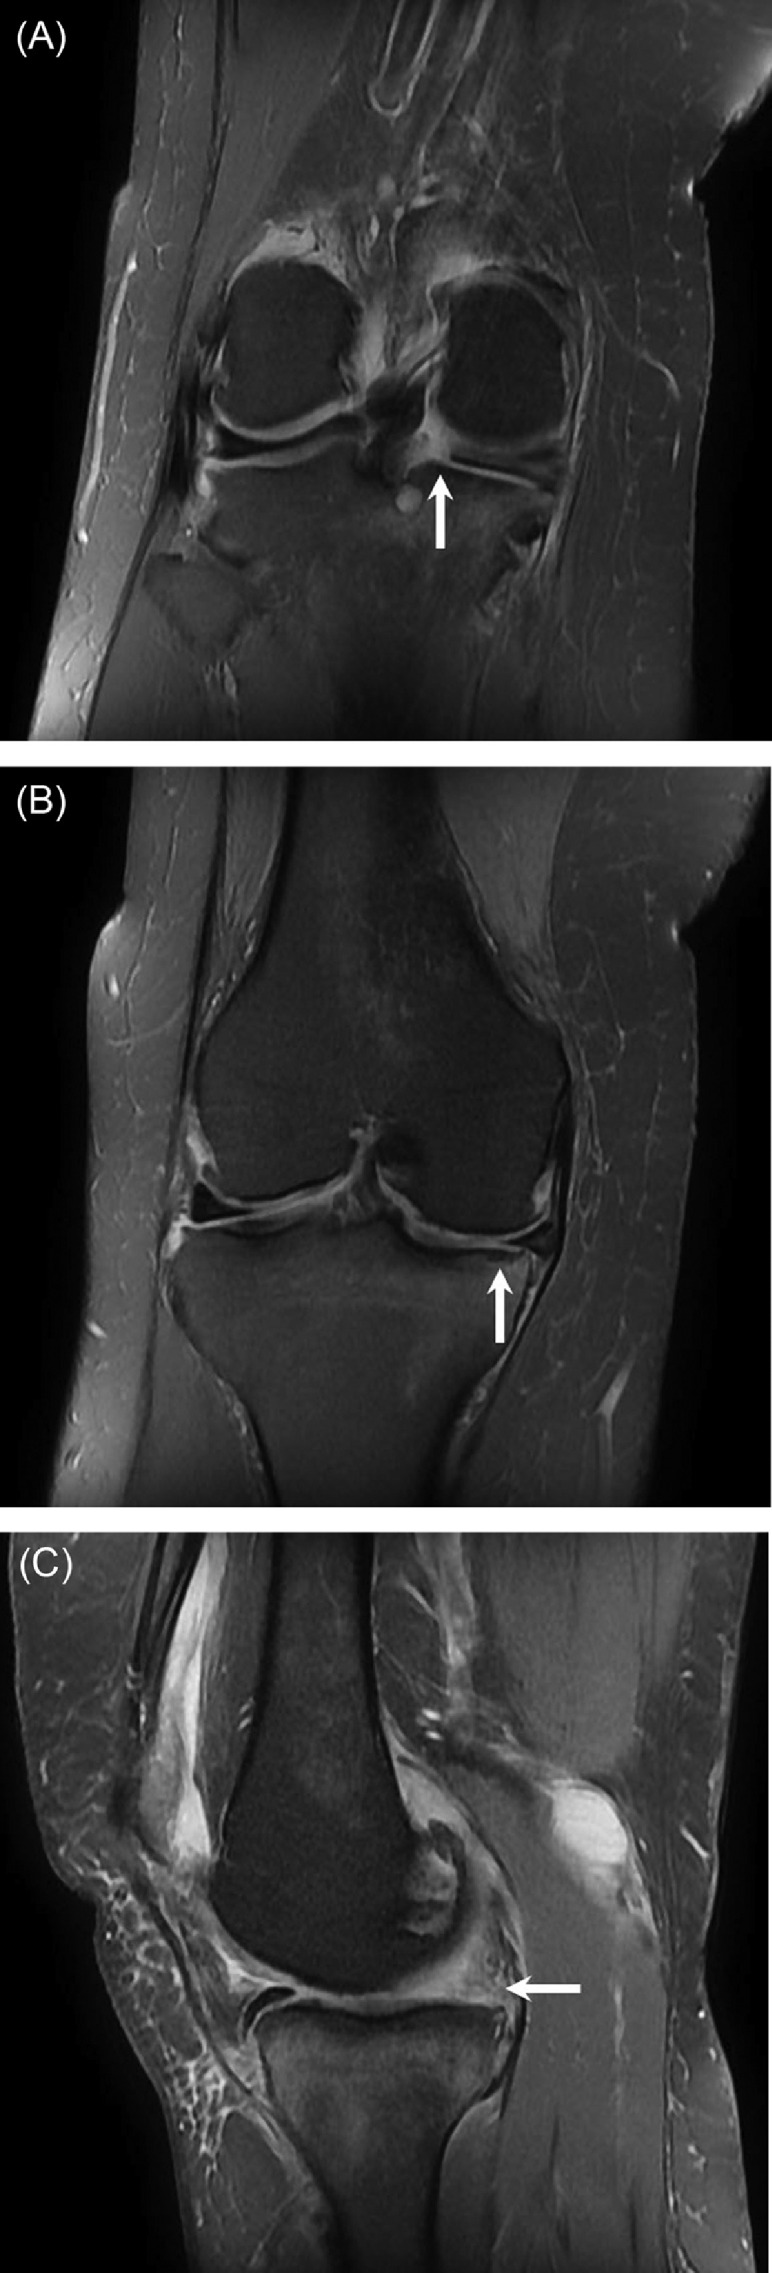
(A) Preoperative MRI. Coronal PD FS MRI image shows a complete radial tear (arrow) of the posterior root of the medial meniscus. (B) Preoperative MRI. Coronal PD FS MRI image shows extrusion of the body of the medial meniscus from the joint space. Partial thickness chondrosis is visible in the medial femoral condyle. There is subchondral marrow edema in the medial tibial plateau with a small area of linear hypointensity suggestive of subchondral insufficiency fracture (arrow). (C) Preoperative MRI. Sagittal PD FS MRI image shows complete absence of the posterior root of the medial meniscus, compatible with a complete root tear (arrow). There is partial thickness chondrosis in the medial femoral condyle, subchondral marrow edema in the medial tibial plateau, partially imaged knee joint effusion and Baker's cyst.
